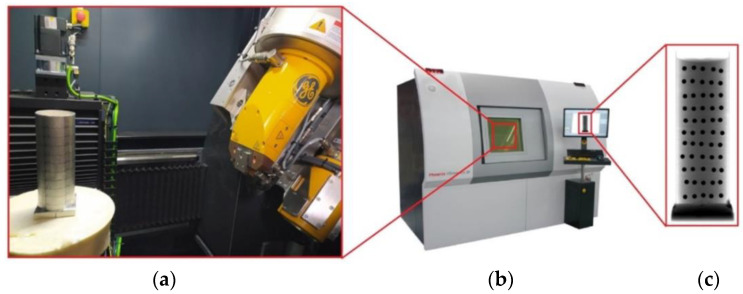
X-ray test stand, (a) setting the die on the rotating X-ray tomograph, (b) General Electric phoenix v|tome|x m X-ray tomograph, (c) X-rayed die.
